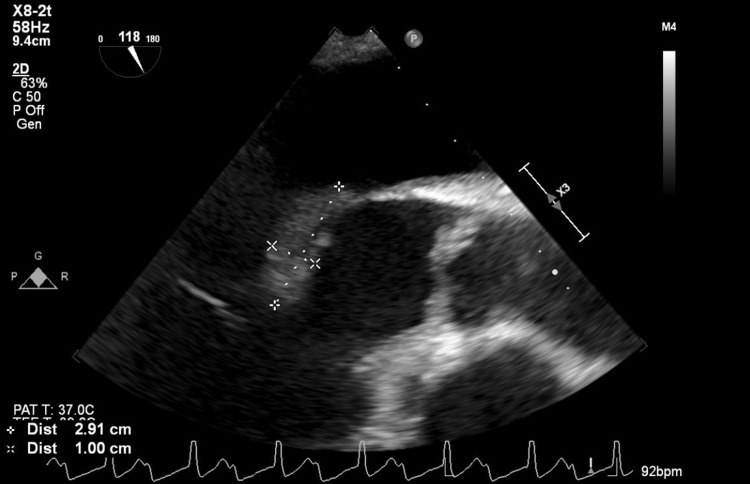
Transesophageal echocardiogram image showing large mitral valve vegetation
